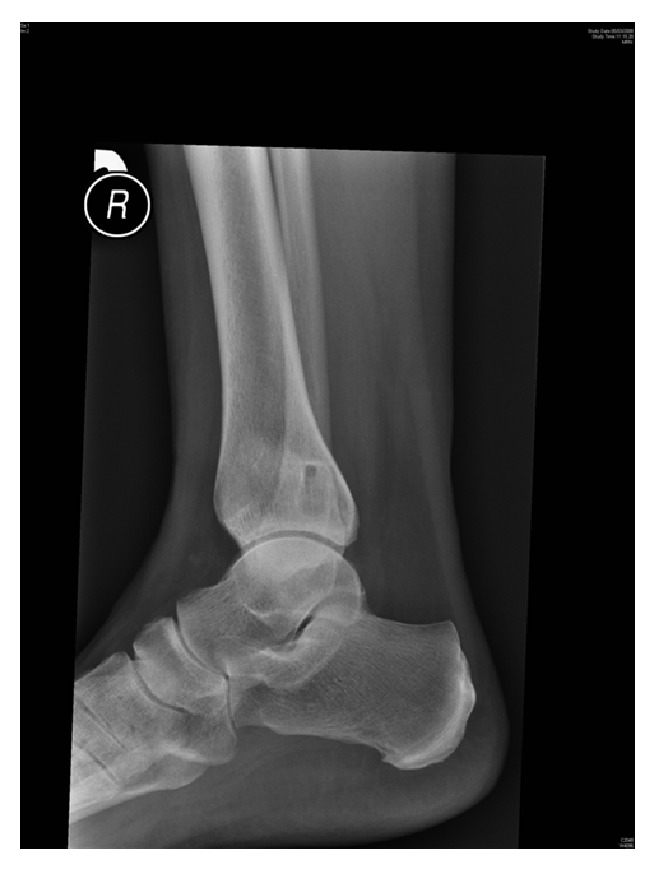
Lateral view X-ray showing reoccurring cystic lesion in distal tibia.
